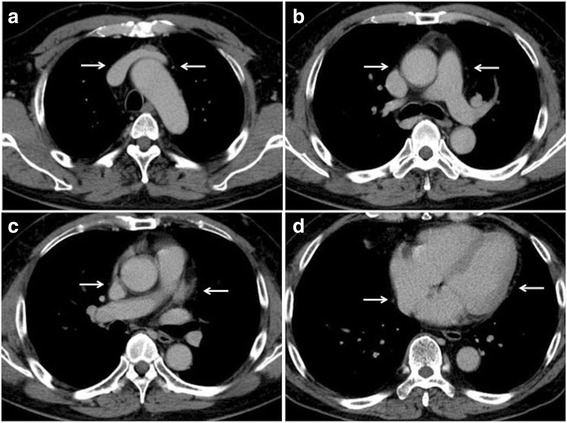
Representative location of the bilateral PCPV, which runs parallel to the phrenic nerves The PCPV on each CT slice is shown as dotted structures (arrows). a On the right side, the phrenic nerve lies laterally to the right brachiocephalic vein and the superior vena cava, while the left phrenic nerve runs laterally to the aortic arch. b-d The bilateral phrenic nerves pass anteriorly to the respective pulmonary hila and inferiorly in a broad vertical plane along the margin of the heart between the parietal pericardium and the mediastinal pleura.
